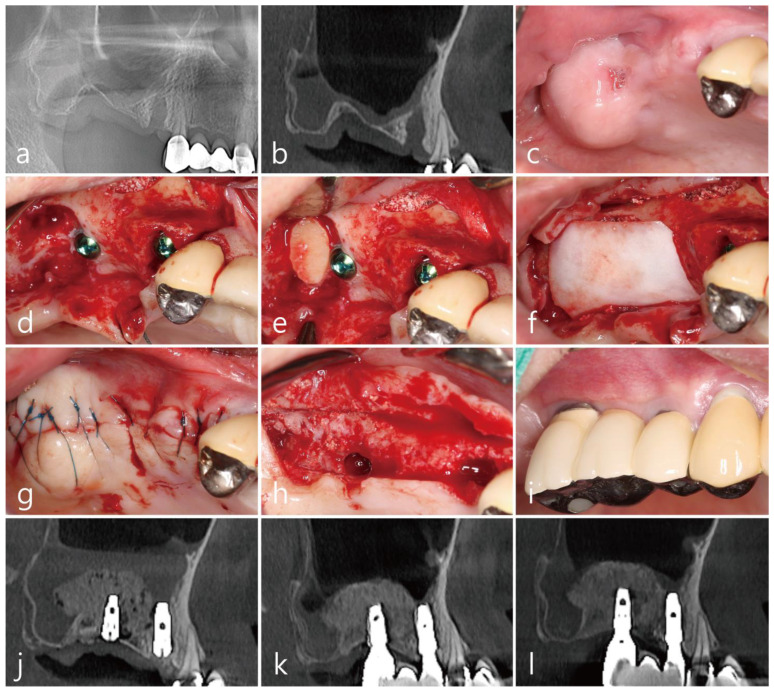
Case 1: (a) preoperative panoramic radiograph; (b) sagittal image of the preoperative CBCT scan showing a severely resorbed bone defect; (c) preoperative clinical view two months after tooth extraction; (d) after the flap reflection, a lateral window was prepared, and the buccal bony window was obtained during the maxillary sinus augmentation; (e) the obtained lateral bony window was passively placed on the distal intraosseous defect of the #17 implant without fixation; (f) the surgical site was covered with a resorbable collagen membrane; (g) the flaps were closed with minimal tension; (h) the uncovering procedure was performed six months after the operation, and the lateral window bone was well incorporated with the adjacent native bone; (i) clinical view at two years after prosthesis delivery; (j) panoramic radiograph immediately after surgery; (k) sagittal image of the CBCT scans after prosthesis delivery; (l) sagittal image of the CBCT two years after prosthesis delivery, where the lateral bony window was well incorporated with the surrounding native bone, and the bone density was increased.
